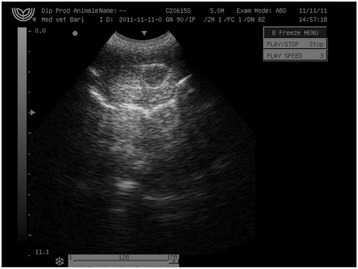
Scrotal ultrasonography after intratesticular injection of CaCl
